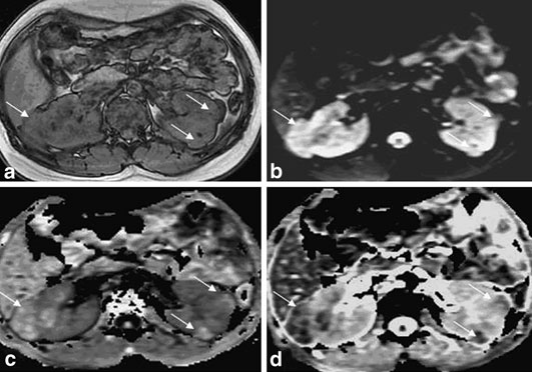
Multiple renal angiomyolipomas in a 16 years old girl. a T1WI in-phase showed irregular prominence of signal image within *right* kidney, and multiple prominent extrarenal semicircular signal intensity within *left* kidney, with ill-defined border. b DWI lesions showed uneven slightly higher signal intensity. c The EADC map showed uneven high signal with a clear boundary, and the lesions were highlighted in the context of gray kidney. d ADC map showed uneven low signal intensity with cluttered kidney background
